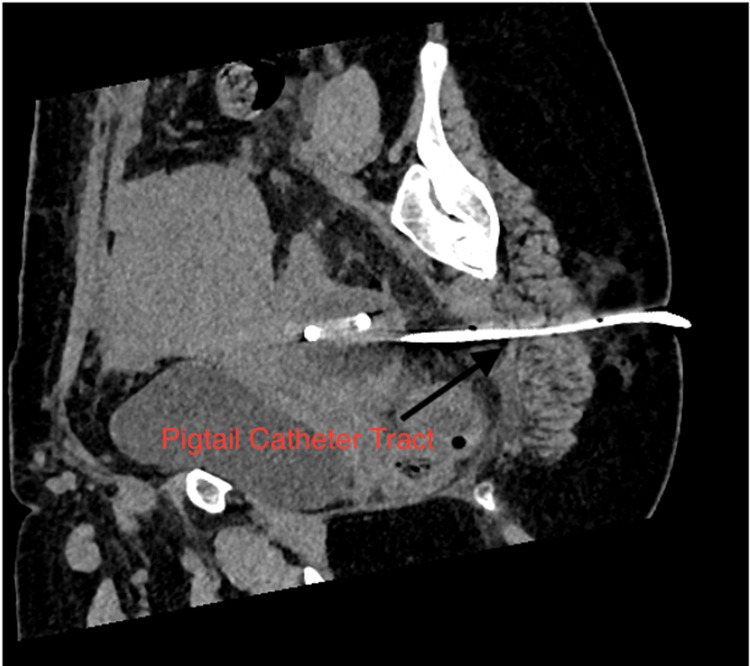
CT scan image showing track of pigtail catheter in sagittal section.
